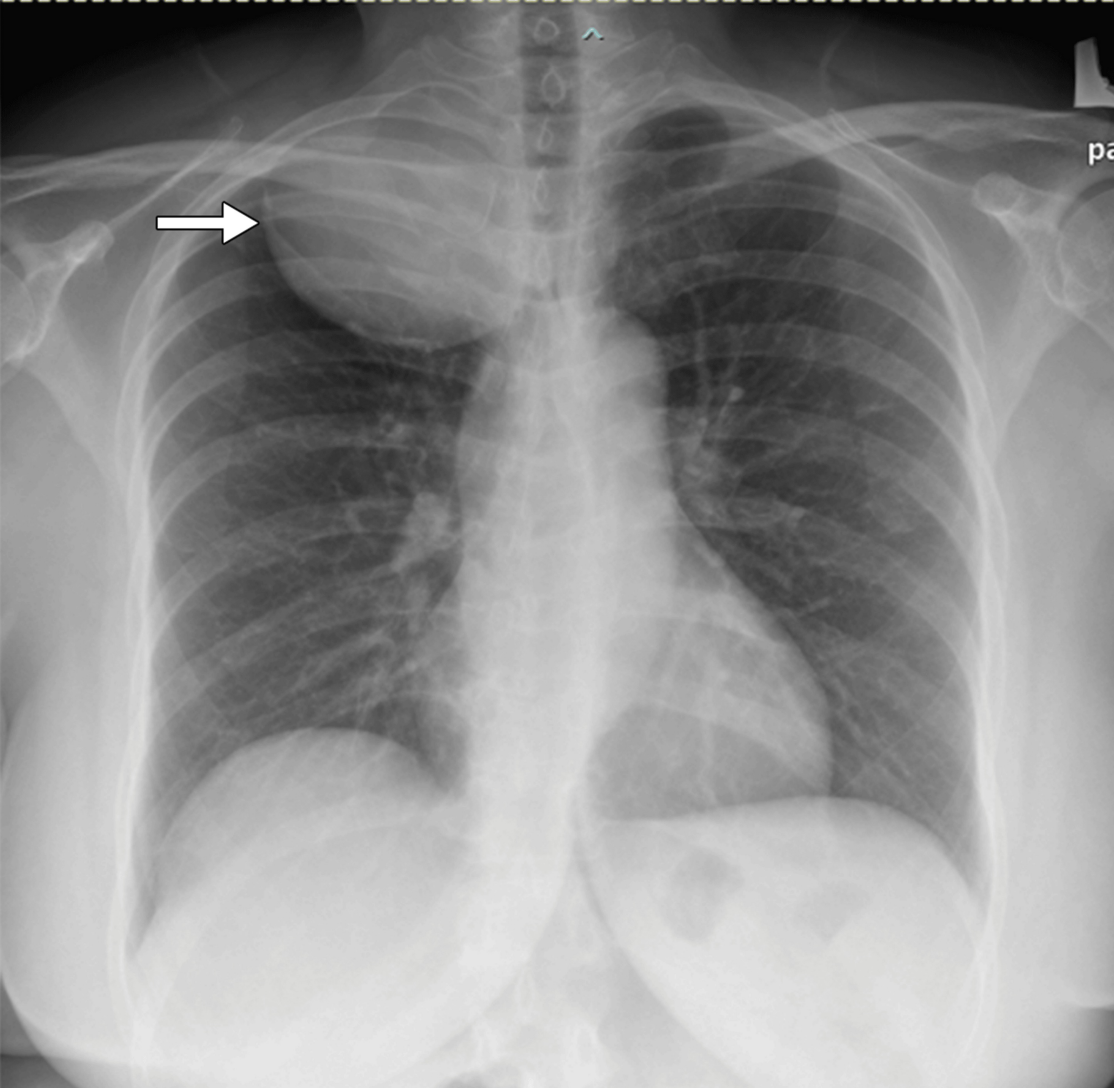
Chest radiography showing the intrathoracic portion of the dumbbell tumour (white arrow).
